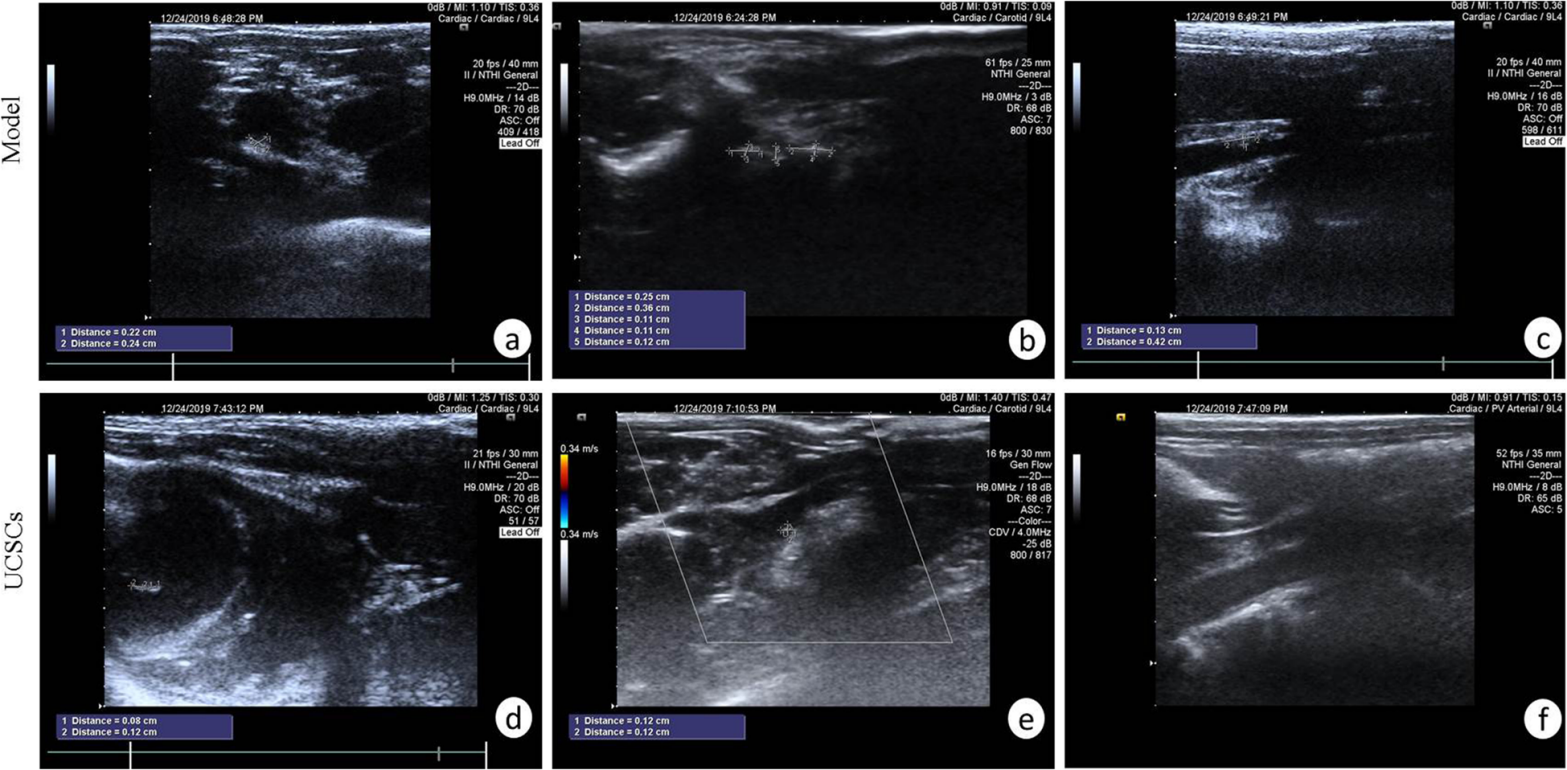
Ultrasonic detection of abdominal aorta and carotid artery. A Plaque in the aortic arch. B Several small plaques at the carotid artery bifurcation. C Plaque in the middle part of the abdominal aorta. D Hyperechoic plaque of aortic valve leaflet. E Small plaques at the carotid artery bifurcation. F Normal abdominal aorta (n = 10 in each group)
